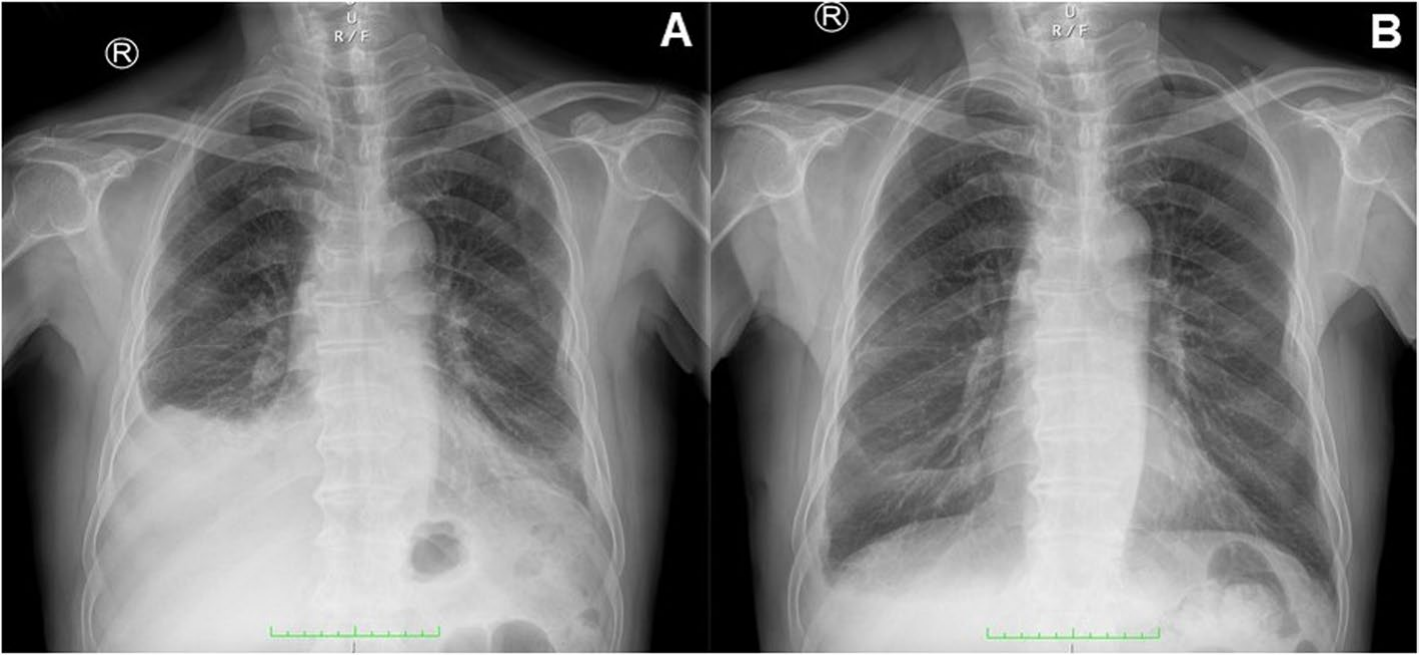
Chest radiography image of the patient
